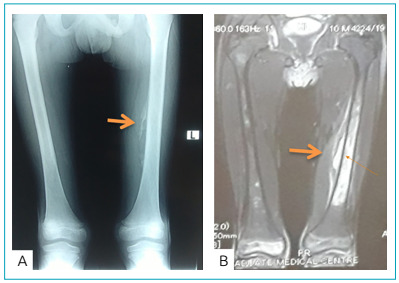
Radiological imaging depicting signs of osteomyelitis. (A) Plain radiograph of left leg indicates mild periosteal reaction and periosteal elevation with sub-periosteal collection and displacement and blurring of fat planes. (B) Magnetic resonance imaging with contrast showing marrow signal abnormality in the mid-shaft diaphysis of the left femur.
